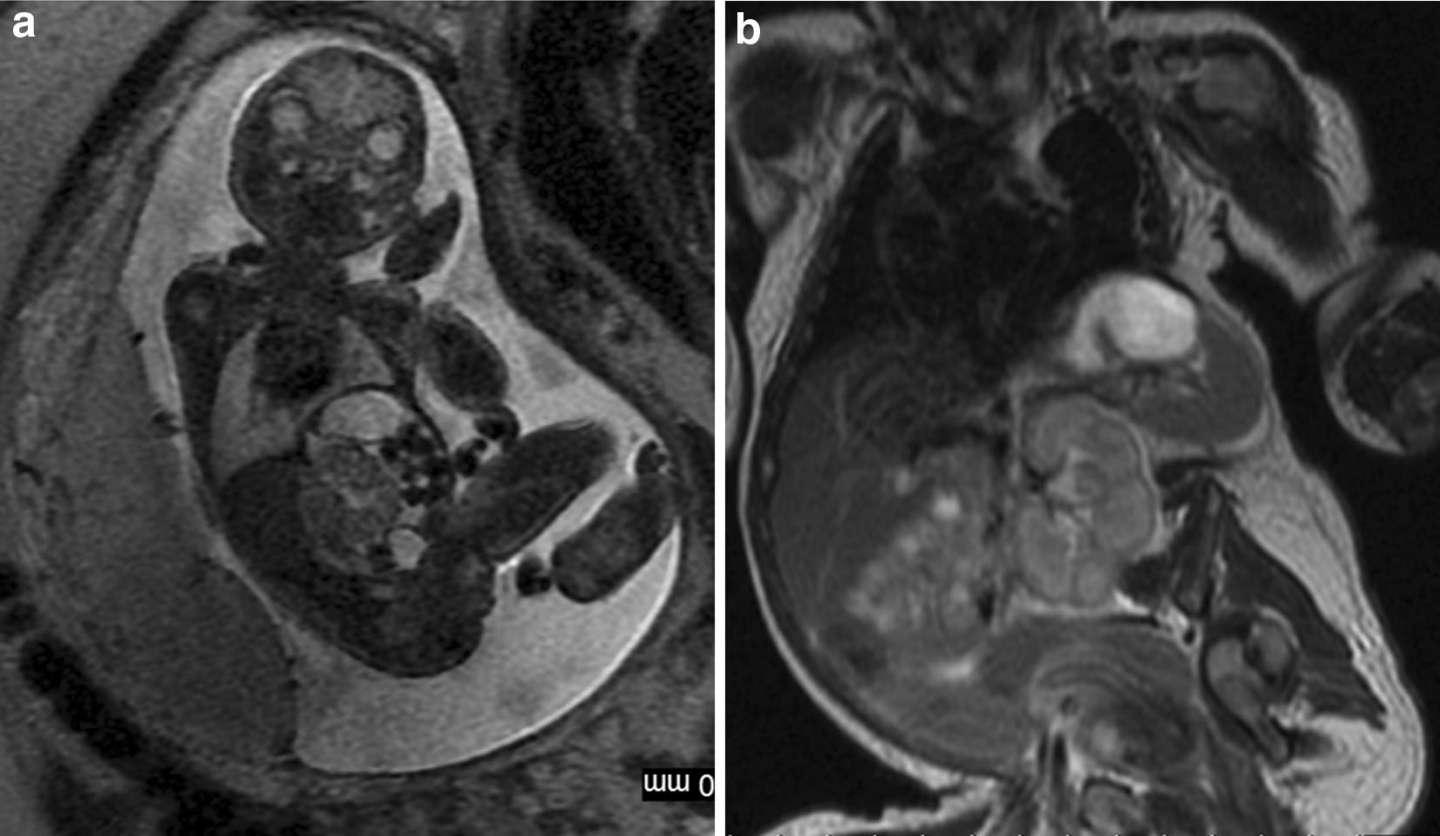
Case no. 1. Single ectopic kidney is seen in both examinations. a Prenatal MRI. T2-weighted image, coronal oblique plane. The uterus was impossible to assess. b Postnatal MRI. T2-weighted image, coronal plane. Unicornuate uterus with a typical *banana shape*
